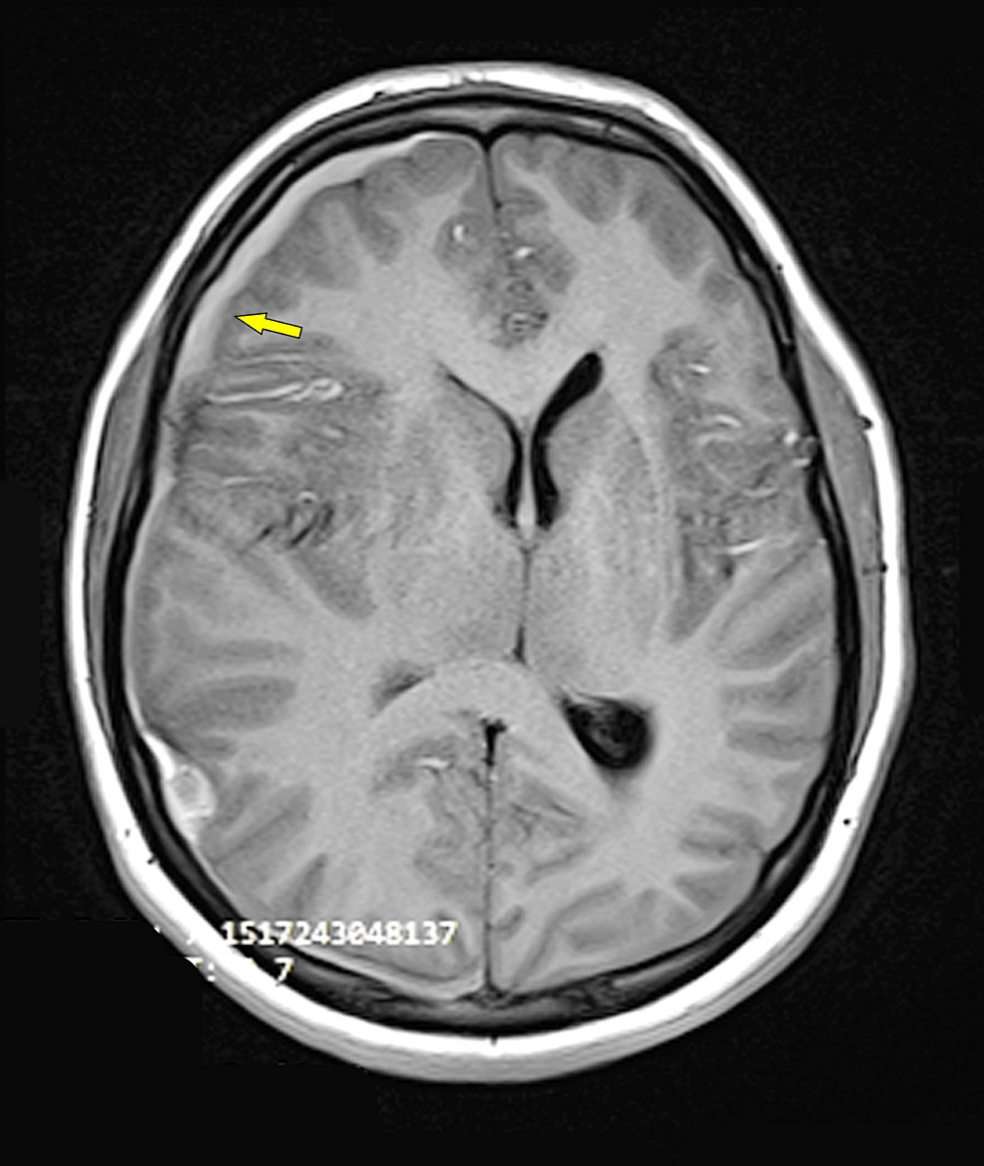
MRI axial T1 weighted image showing extra axial collection (subdural hemorrhage) along with right convexity (yellow arrow). Mass effect is seen compressing upon the right ventricle with a midline shift of 8.4 mm towards the left side.
